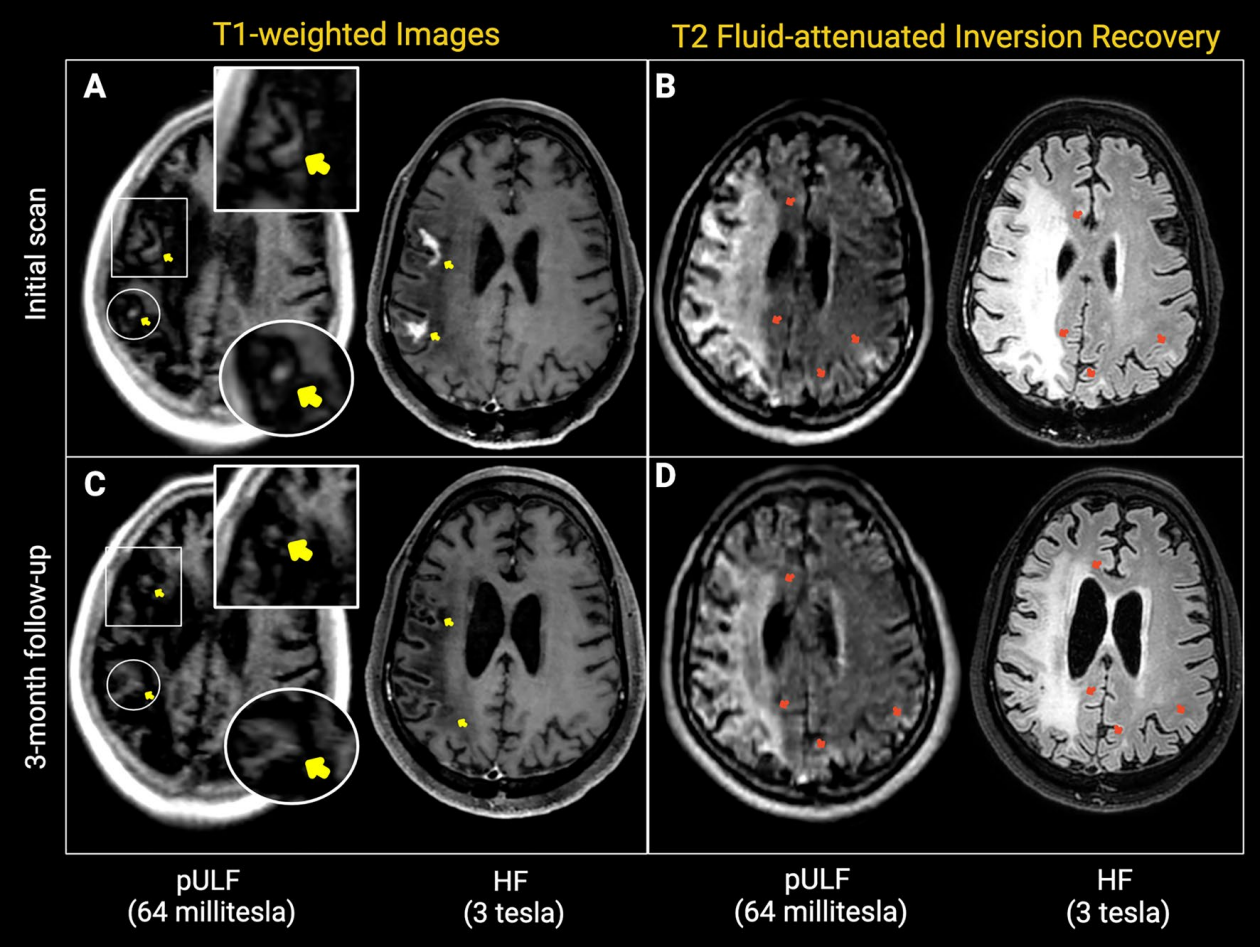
Contrast enhancement and its resolution on follow up scan. 49-year-old man with PML and underlying HIV. Initial (panels A, B) and 3-month follow up (panels C, D) pULF-MRI acquired on the same day following HF-MRI are shown. Initial scans showing contrast enhancement on T1W images (yellow arrows; panel A) and extensive white matter lesion in the right cerebral hemisphere and left parietal lobe on T2-FLAIR images (red arrows; panel B) at both magnetic strength fields. On 3-month follow up, contrast enhancement areas are not visible on T1WI (yellow arrows; panel C), and lesion regression with ex vacuo enlargement of the nearby ventricle and sulci on T2-FLAIR (red arrows; panel D), are noted at both magnetic fields
